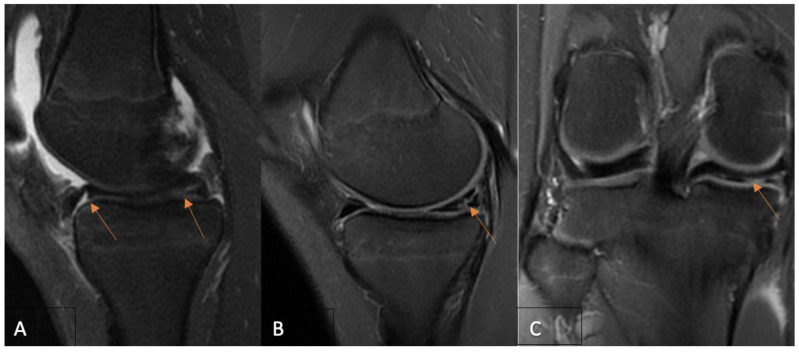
(A) A 14-year-old girl’s preoperative sagittal PD-FSE, FS MR imaging demonstrates (arrows) a medial meniscus tear of the bucket-handle type. Fourteen-month postoperative coronal (B) and sagittal (C) PD-FSE FS MR images establish (arrows) an unhealed medial meniscus with signal changes extending within the joint space—MRI grade 3 according to Crues and Stroller grading. MR—magnetic resonance; FSE—fast spin echo; FS—fat saturation.
